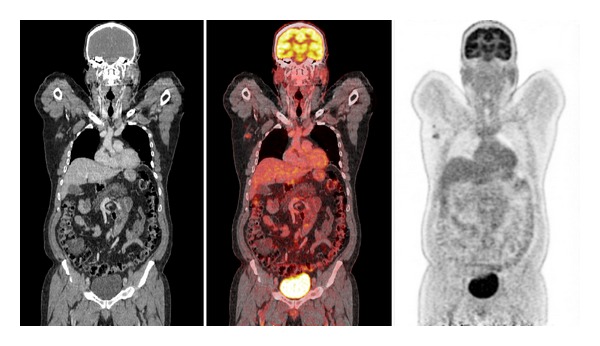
59-year-old man with right scapular melanoma (Breslow 2.4 mm) undertaking PET/CT scan for initial staging. Coronal view with pathologic right axillary nodes of 1.4 cm and SUVmax 7.7.
